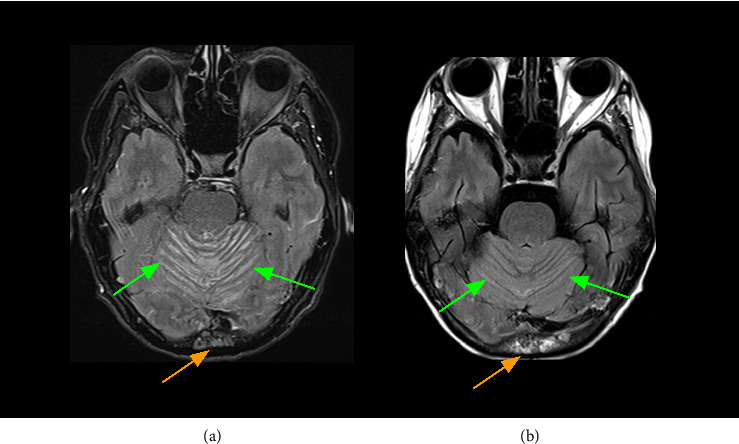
Improvement in cerebellar sulcal enhancement. Post gadolinium axial T2 fluid-attenuated inversion recovery (FLAIR) images. (a) Before treatment with teclistamab, deep resolve boost (DRB): yes, repetition time (TR): 9400.0 ms, echo time (TE): 81.0 ms, and echo train length (ETL): 21. (b) After treatment with teclistamab, DRB: no, TR: 9000.0 ms, TE: 83.0 ms, and ETL: 17. Initial diffuse leptomeningeal enhancement along the cerebellar folia shows dramatic improvement following treatment (green arrows). Orange arrows demonstrate one of the several osseous lesions due to multiple myeloma.
